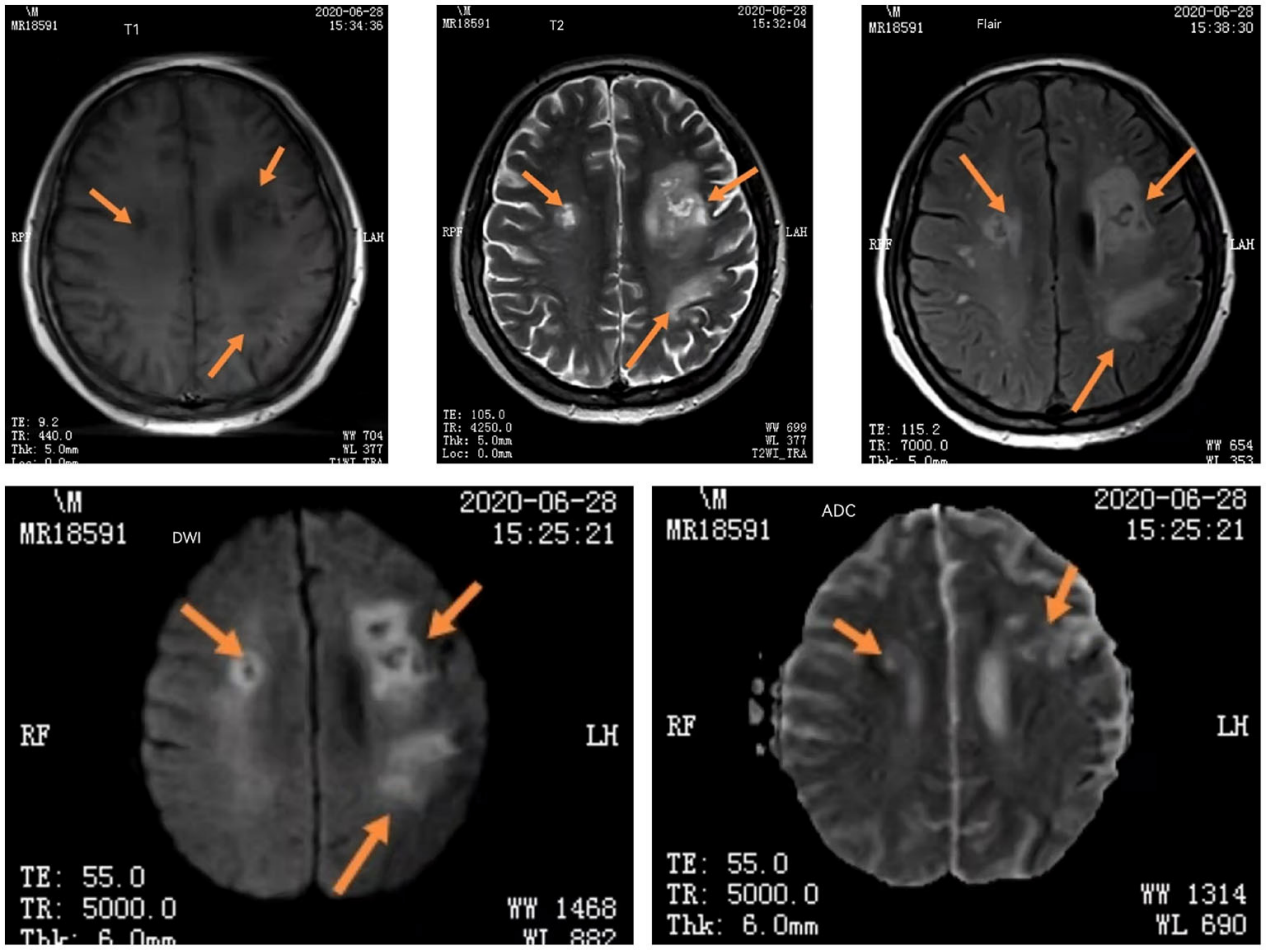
The iconography results of re-examinations of the patient. Head MRI (T1, T2, FLAIR, DWI, ADC) showed bilateral frontal and parietal cortex necrosis and meningoencephalitis. The arrows are showing changes in head MRI of bilateral frontal and parietal cortex necrosis and meningoencephalitis.
